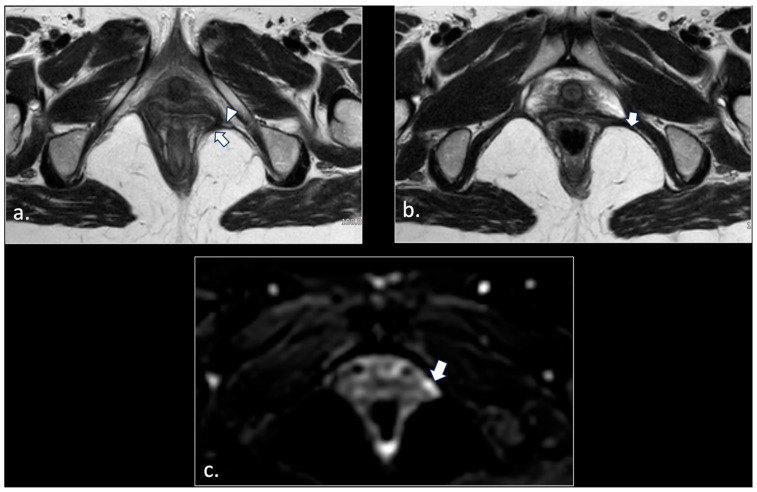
(a) Axial T2-weigthed section through the distal Alcock’s canal shows an atrophy of the left pubo-coccigeal muscle (arrow) and paravaginal fibrosis on the same side. (b) A more cranial section shows with better advantage the paravaginal fibrosis (arrow); (a) the wall of the vagina is stretched (arrowhead). (c) b600 DWI section, acquired at the same level of (b), shows a strong hyperintensity and enlargement of the left pudendal nerve (arrow).
